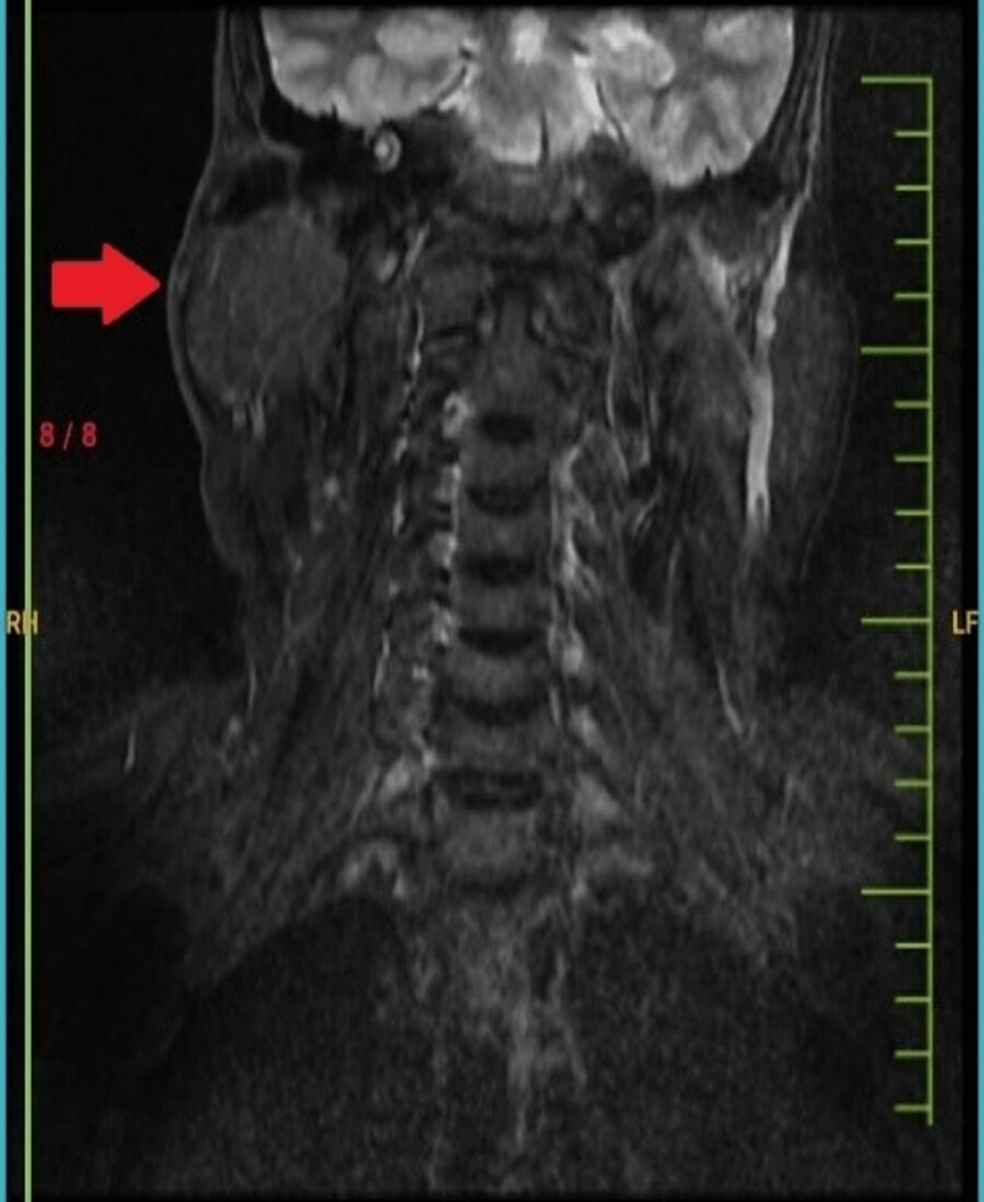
An MRI of the posterior cervical region with an arrow showing enhanced mass.
